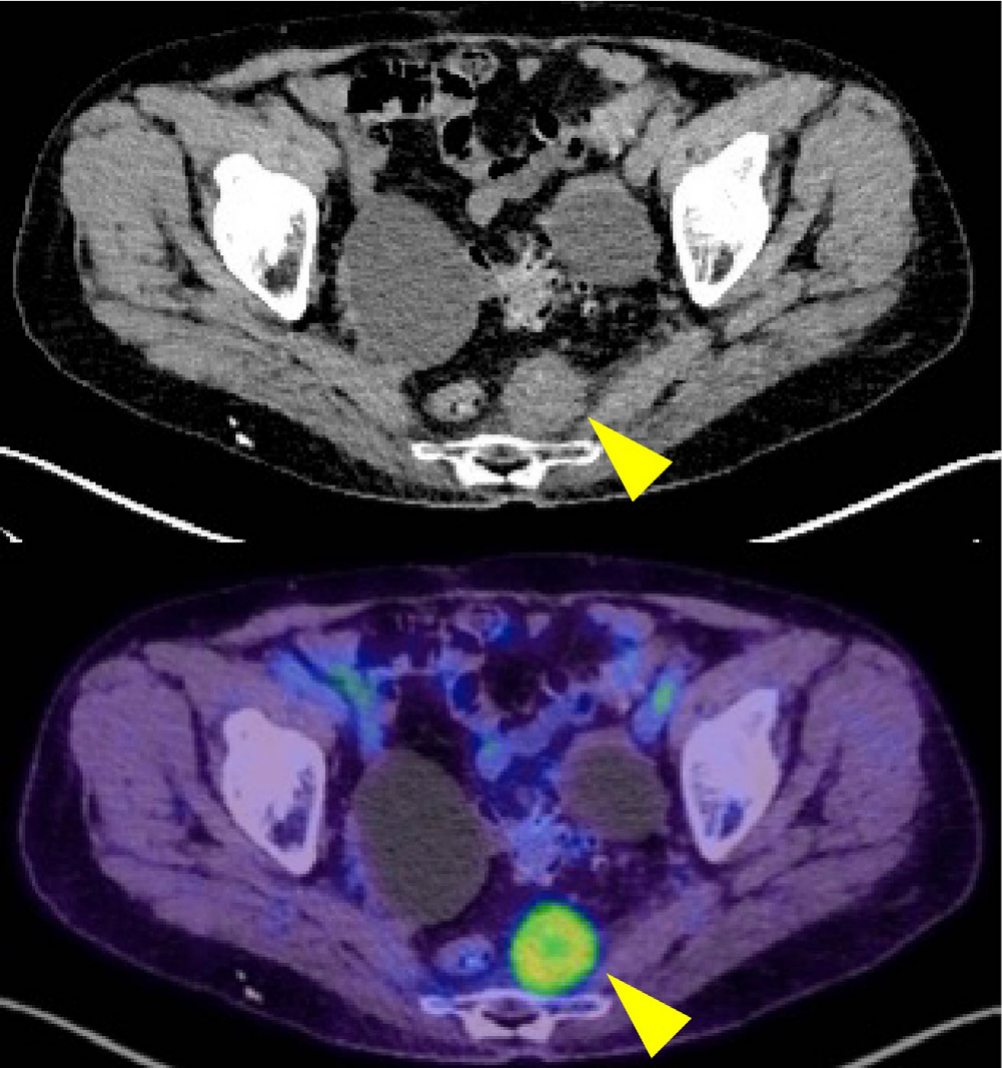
Contrast-enhanced CT and positron emission tomography/CT images. A 3-cm-sized lymph node within the mesorectum (*arrowhead*) was found on the left side.
